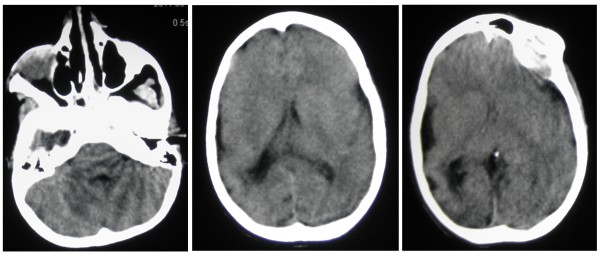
The computerized tomographic (CT) scan of affected individual from family MCP67. The axial CT scan depicts ill defined gryal and nuclei pattern in the affected individual (IV-5) of family MCP67 with homozygous nonsense *WDR62 *mutation.
